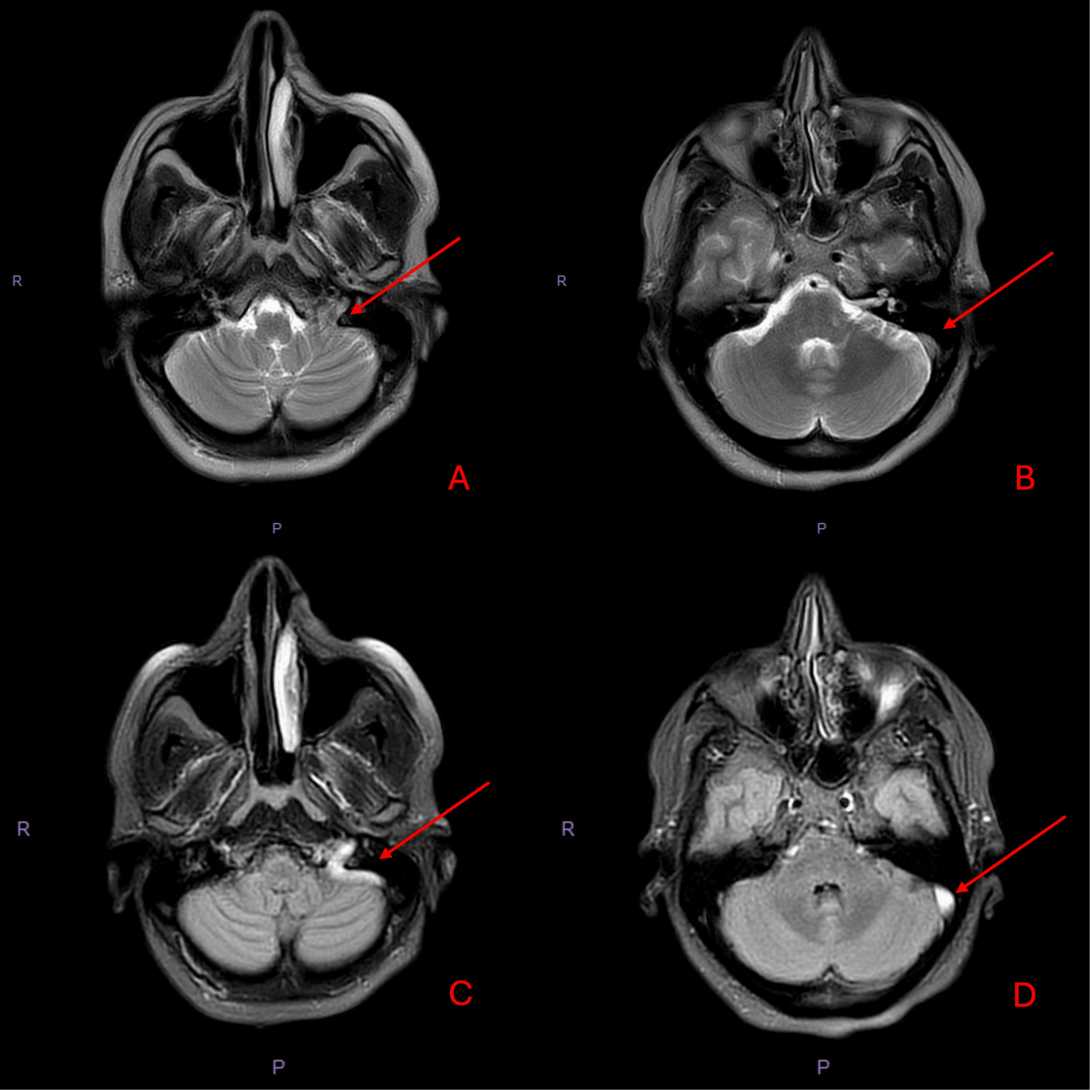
MRI head on admission with equivocal appearances A: T2 MRI with equivocal appearances in the sigmoid sinus (arrow). B: T2 MRI with equivocal appearances in the transverse sinus (arrow). C: FLAIR MRI with equivocal appearances in the sigmoid sinus (arrow). D: FLAIR MRI with equivocal appearances in the transverse sinus (arrow). MRI: magnetic resonance imaging; FLAIR: fluid-attenuated inversion recovery
